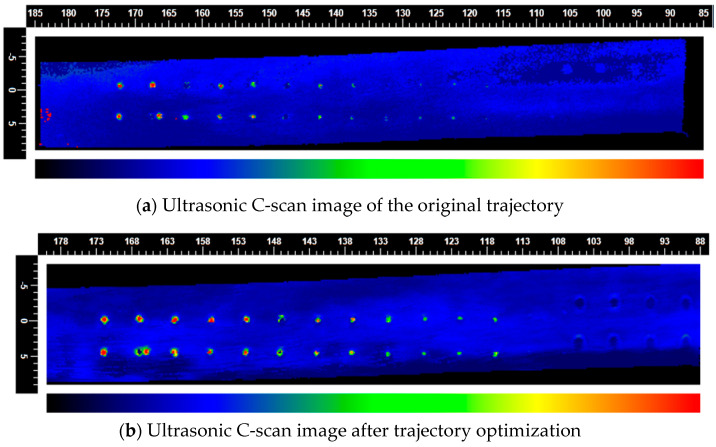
Ultrasonic C-scan image of engine blade.
